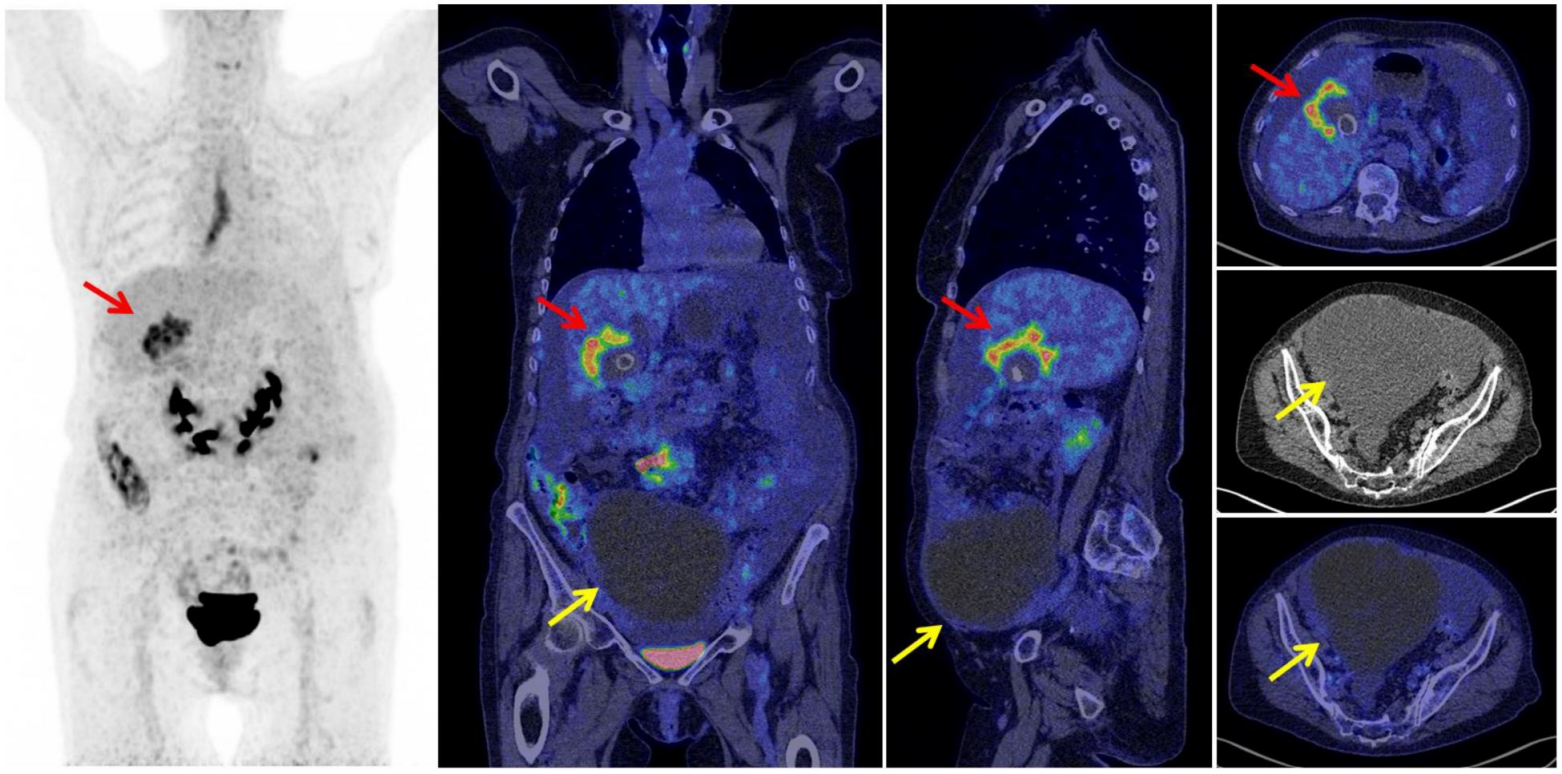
A 73-year-old woman was referred by her general practitioner due to weight loss and abdominal pain. She was examined via ultrasonography, which revealed a multichambered cyst in the pelvis measuring 20×11 cm. She was referred to the Department of Gynaecology, where she underwent an ^18^F-fluorodeoxyglucose PET CT (FDG PET/CT) scan. FDG PET/CT revealed a primary tumour located in the gallbladder (red arrow) with cystic metastasis to the ovary (yellow arrow). The SUV_max_ of the ovarian metastasis was 2.27.
